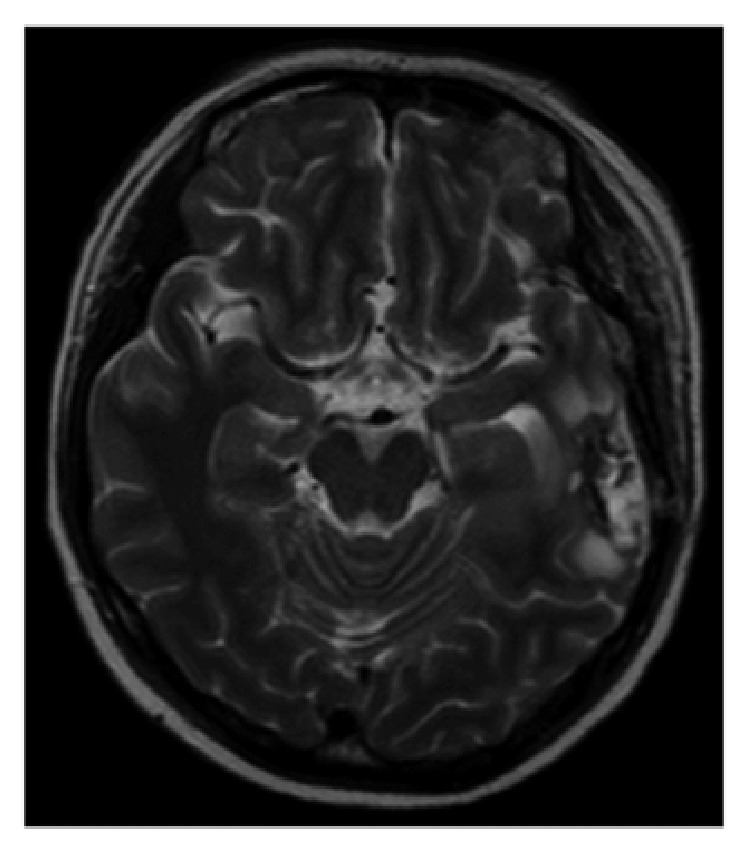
Brain magnetic resonance imaging (horizontal) indicating the absence of the metastatic tumor after urgent craniotomy and chemoradiation.
